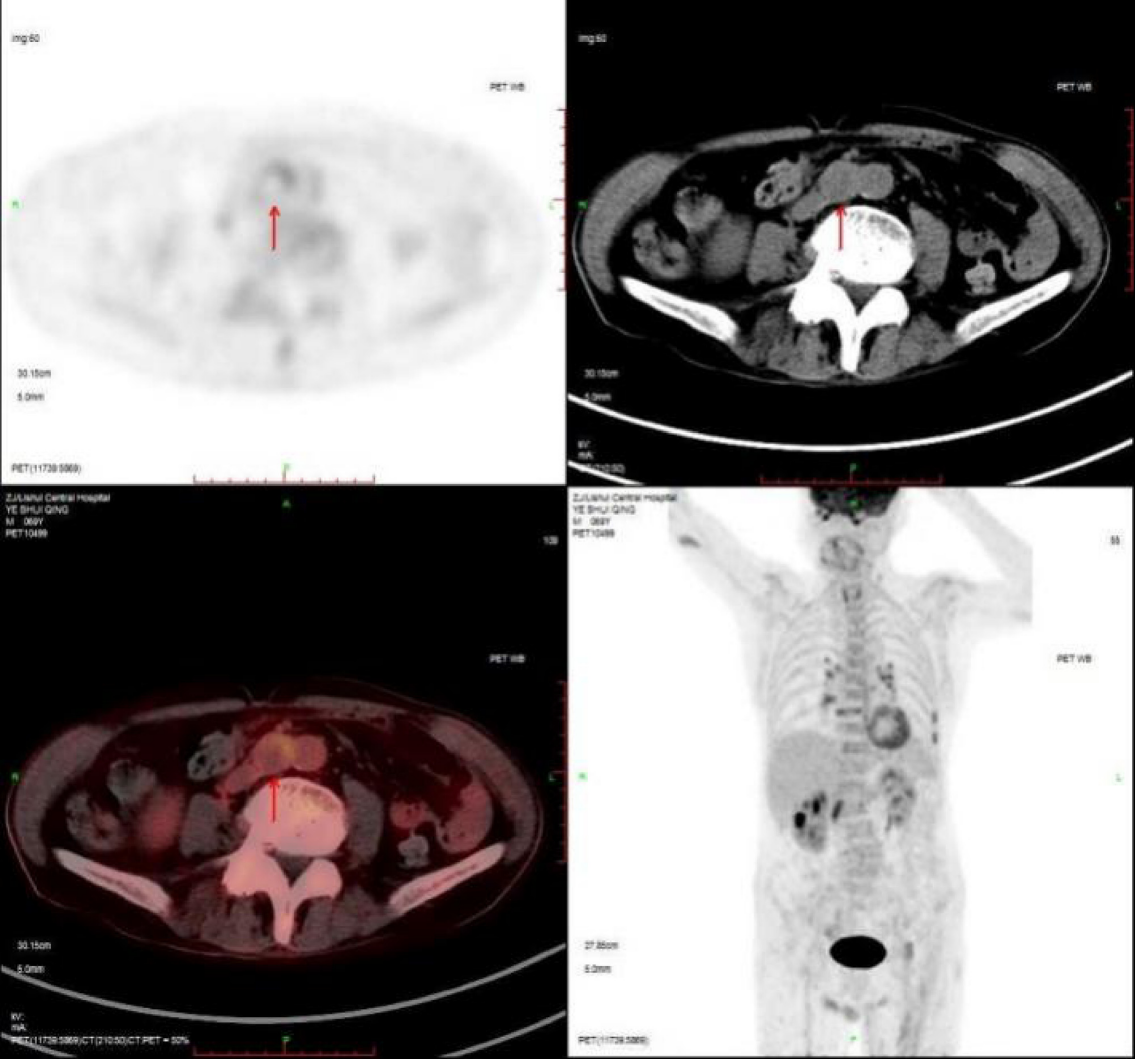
PET-CT scan revealed an isointense mass with a clear boundary near the abdominal aorta with mild uptake of the mass (red arrow).
